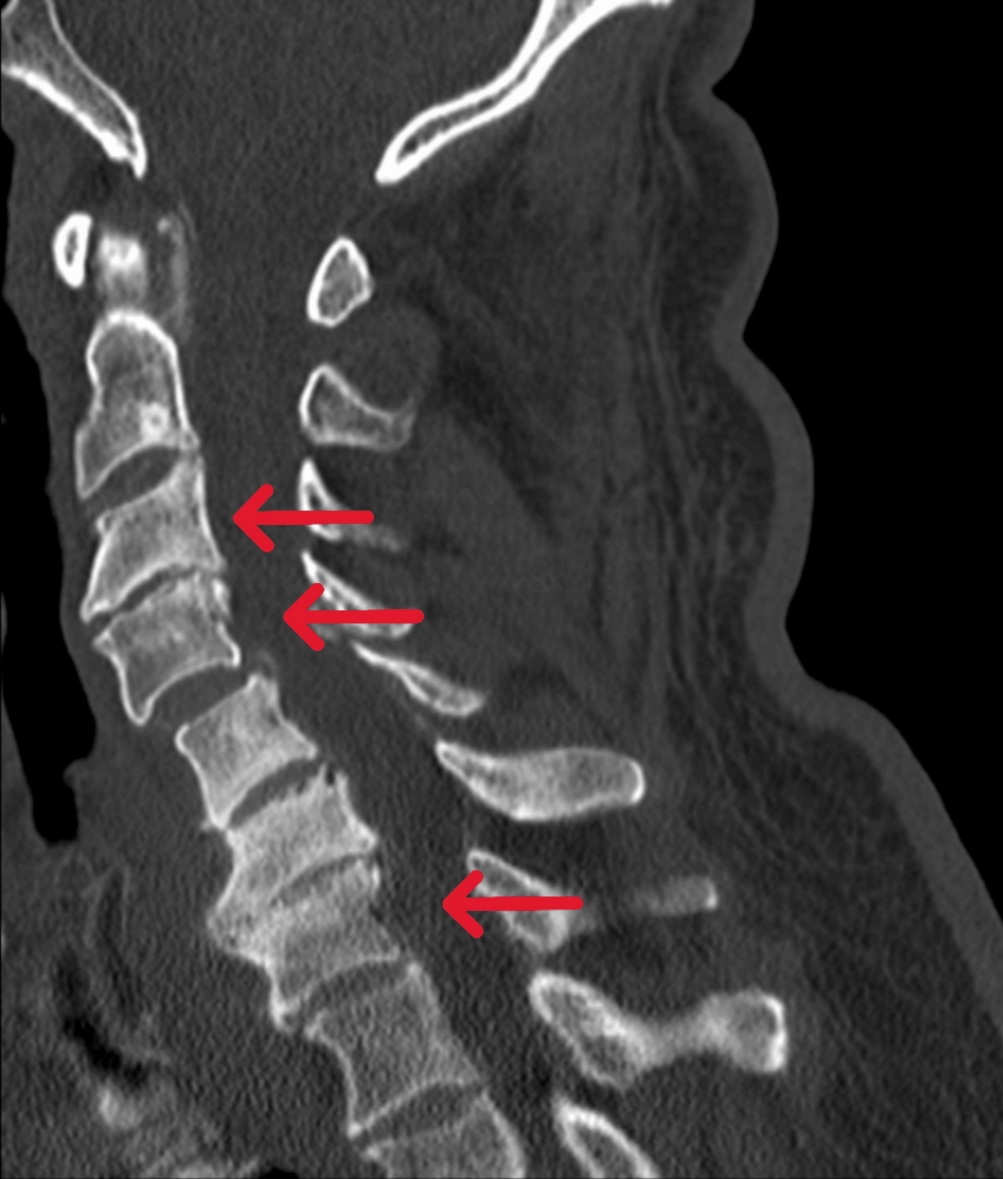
Cervical CT scan Cervical CT scan (sagittal plane) depicting C3 retrolisthesis, C4, and C7 anterolisthesis.
